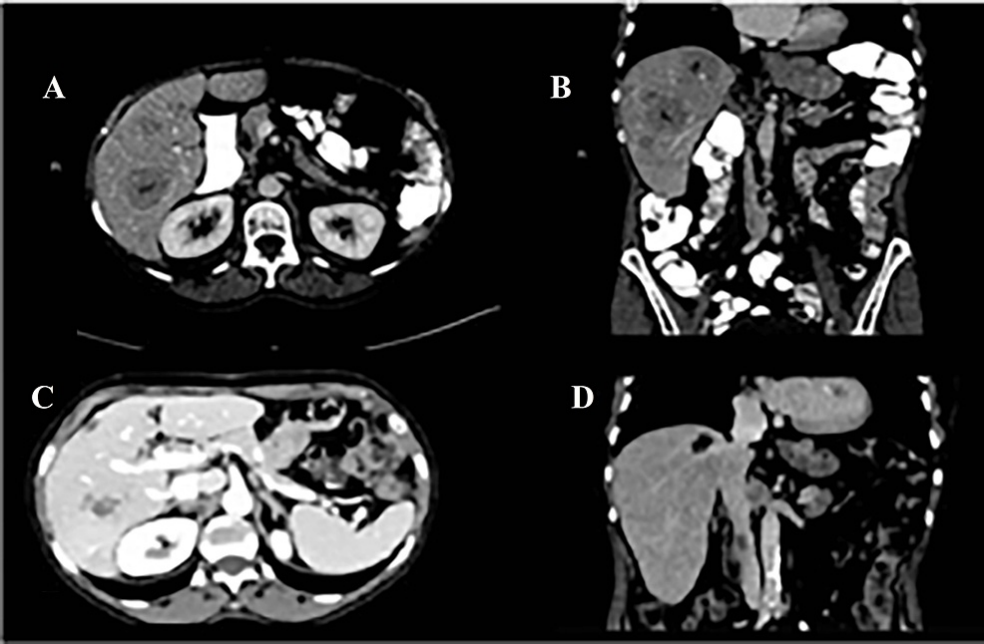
Abdominal CT before (A, axial view; B, sagittal view) and after (C, axial view; D, sagittal view) four months of treatment with pembrolizumab
